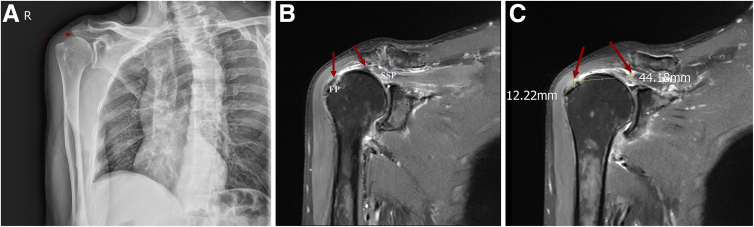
(A) Radiographic examination of the right shoulder joint in the anteroposterior view shows narrowing of the subacromial space, suggesting subacromial impingement syndrome. (R, right.) (B, C) Coronal magnetic resonance imaging of the right shoulder joint shows the residual tissue of the torn supraspinatus tendon (SSP) on the right side (red arrows). The images reveal a massive SSP tear, with the distal tendon stump being retracted medially and of poor tissue quality. (FP, footprint.)
